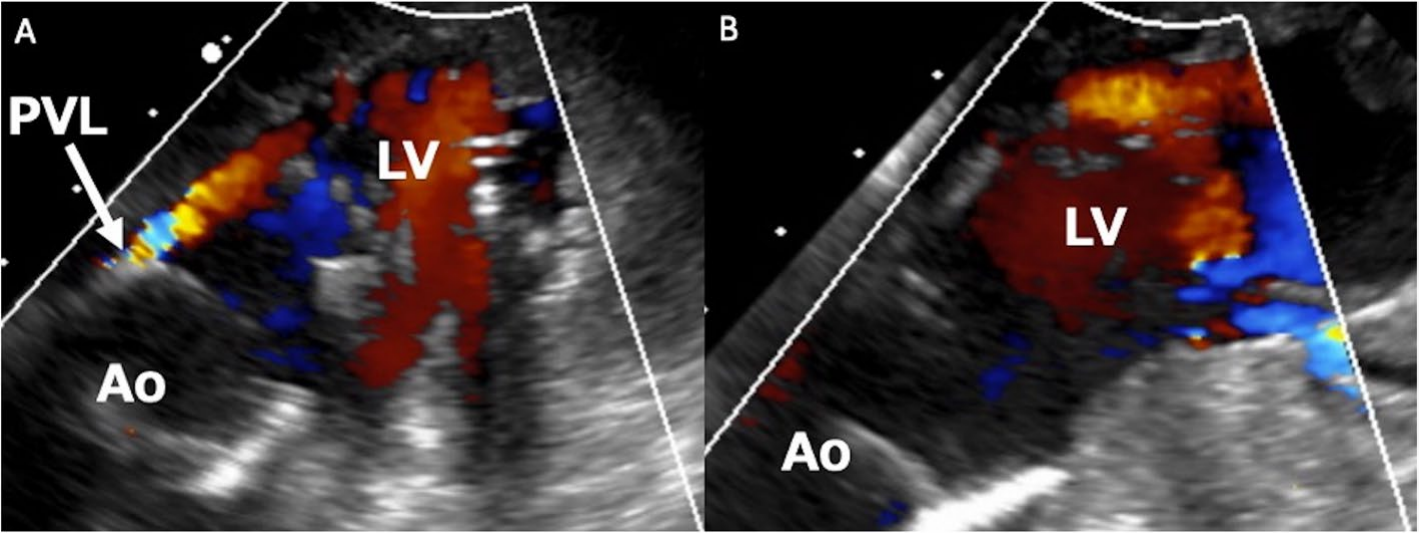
Intraoperative TEE in Case 1. Intraoperative transesophageal echocardiography in Case 1 revealed (A) a moderate paravalvular leak at the left-noncoronary commissure side, and B the leakage resolved after re-ballooning the sealing frame
